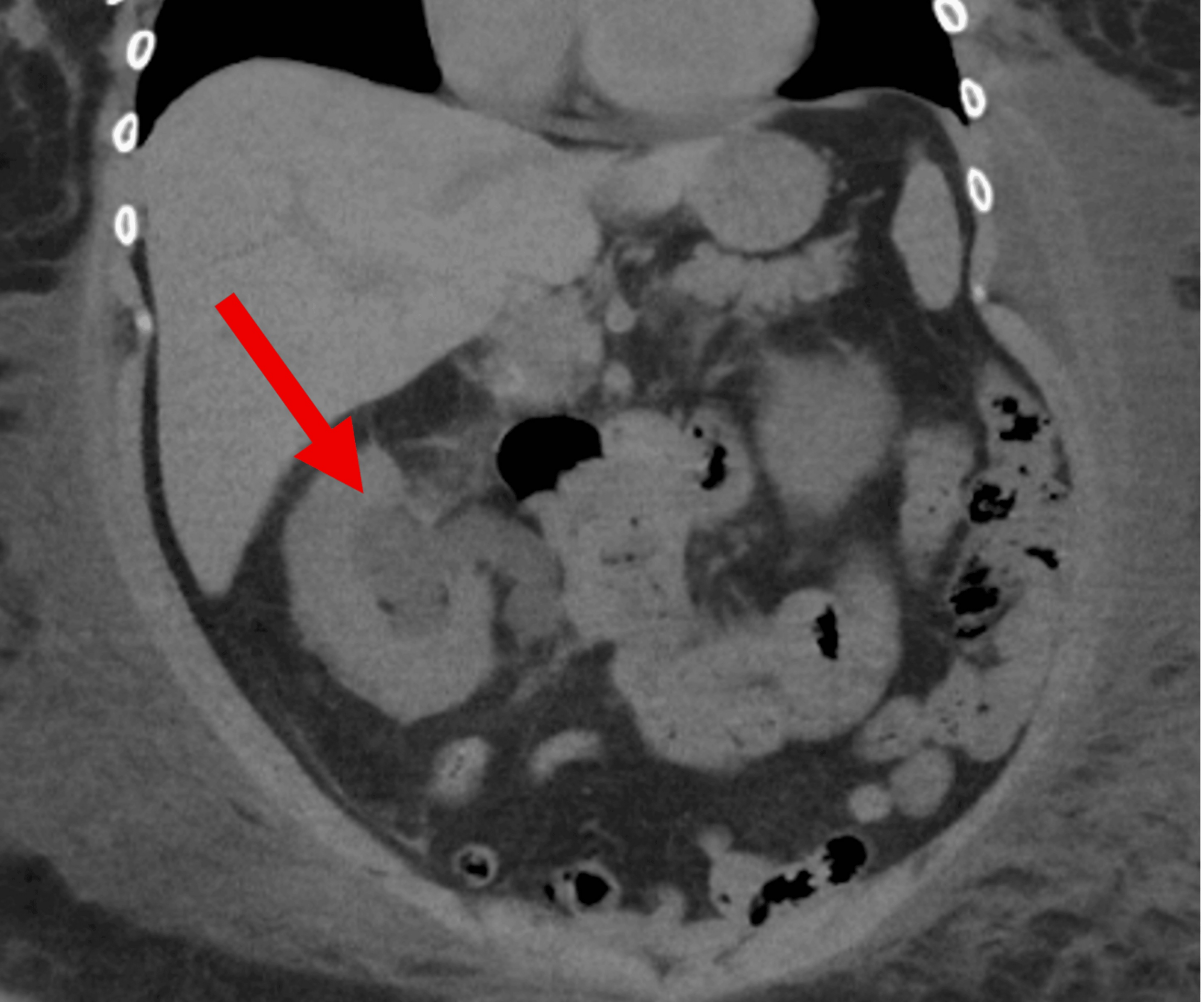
CT of the abdomen and pelvis (coronal) without contrast demonstrating right-sided hydroureteronephrosis (red arrow). CT: computed tomography
